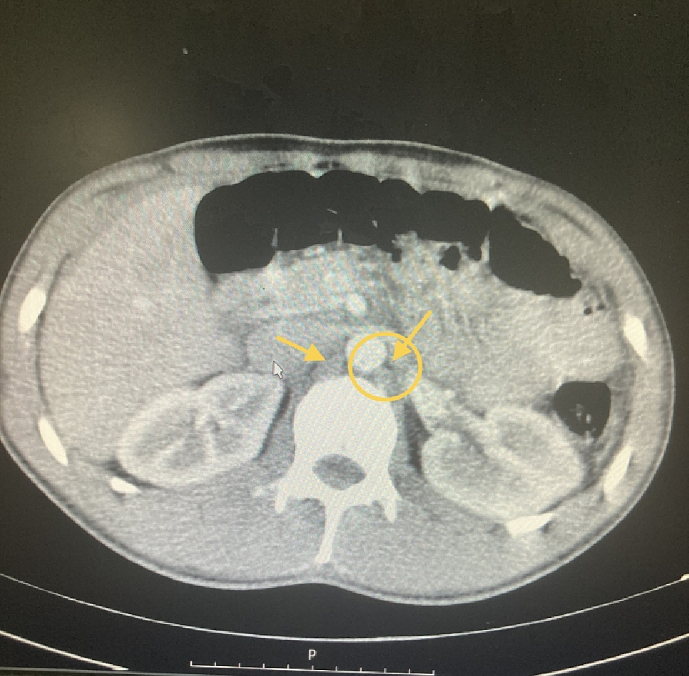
Abdominal CT shows the para-aortic lymph nodes.
